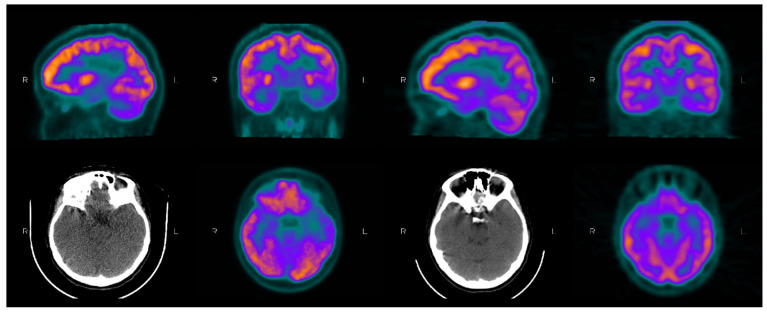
Representative preoperative FDG-PET/CT images from patients who underwent left and right anterior temporal lobectomy. The left panel shows left temporal hypometabolism in a patient who underwent left anterior temporal lobectomy, whereas the right panel shows right temporal hypometabolism in a patient who underwent right anterior temporal lobectomy.
